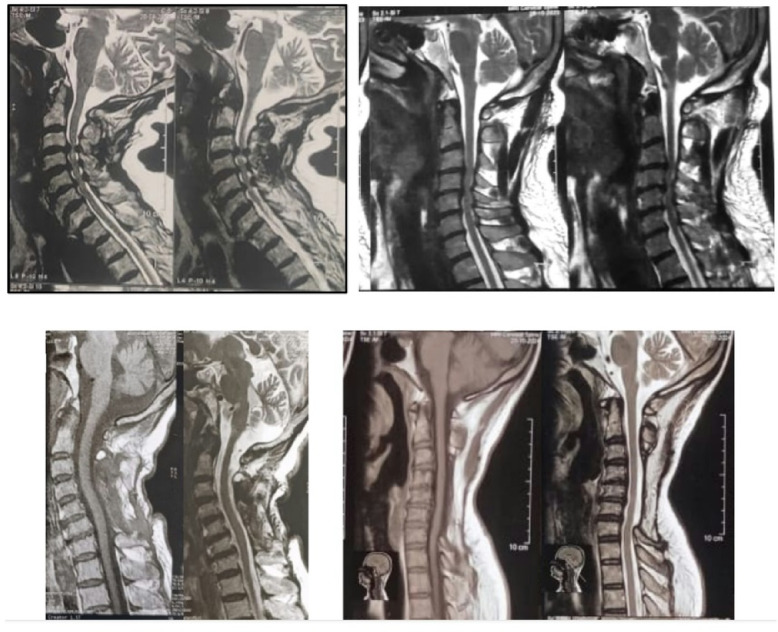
Preoperative and follow-up MRI. Preoperative (upper row) and follow-up (lower row) MRI images. Multi-level spinal cord compressions with T2-hyperintense cord signal noted in the preoperative images. Postoperative follow-up images showing adequate spinal cord decompression, and modest kyphotic progression after multilevel laminectomy.
